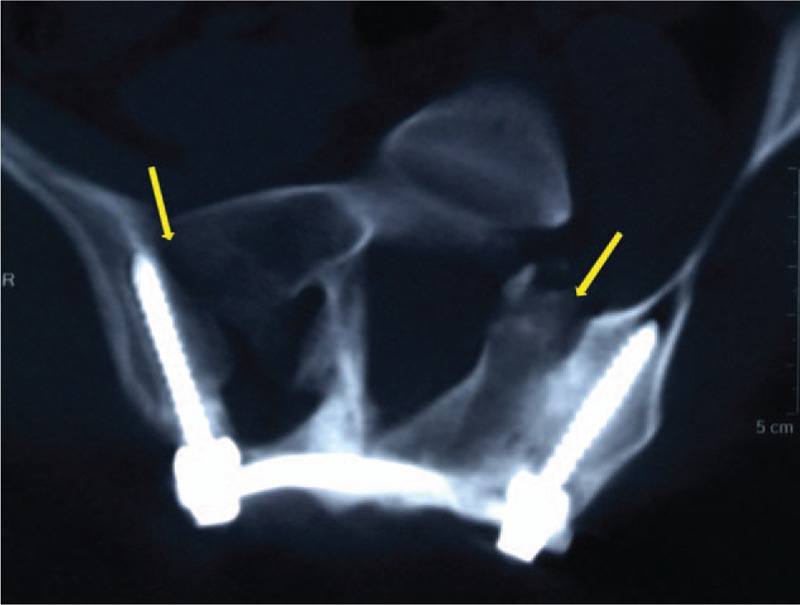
CT showed that complete joint fusion at bilateral sacroiliac joints at the fifth year of follow up. CT = computed tomography.
